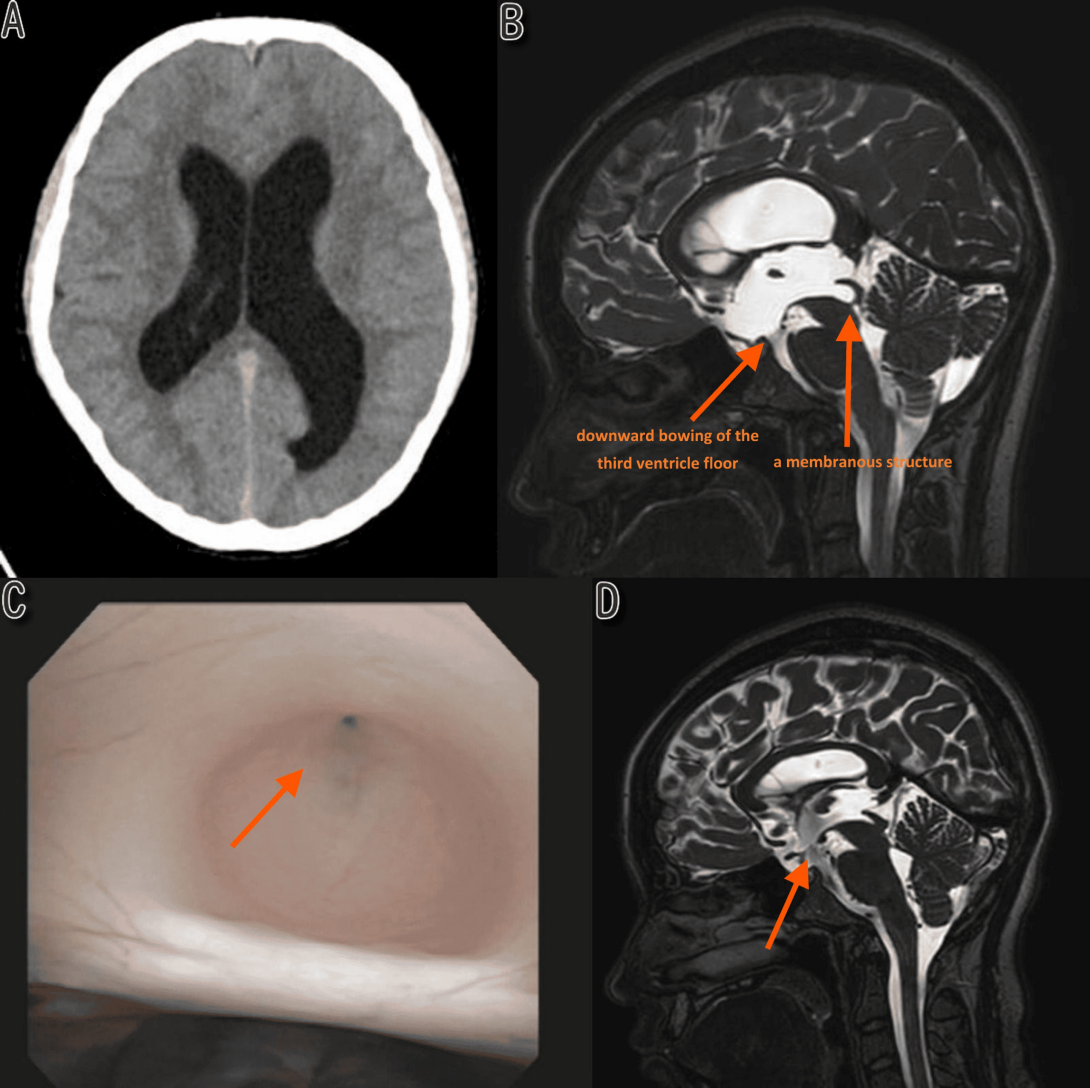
Case 1: (A) Preoperative CT. (B) Preoperative MRI T2WI. (C) Intraoperative endoscopy showing membranous occlusion. (D) Postoperative MRI at 12 months showing stoma patency CT: computed tomography; MRI: magnetic resonance imaging; T2WI: T2-weighted imaging
